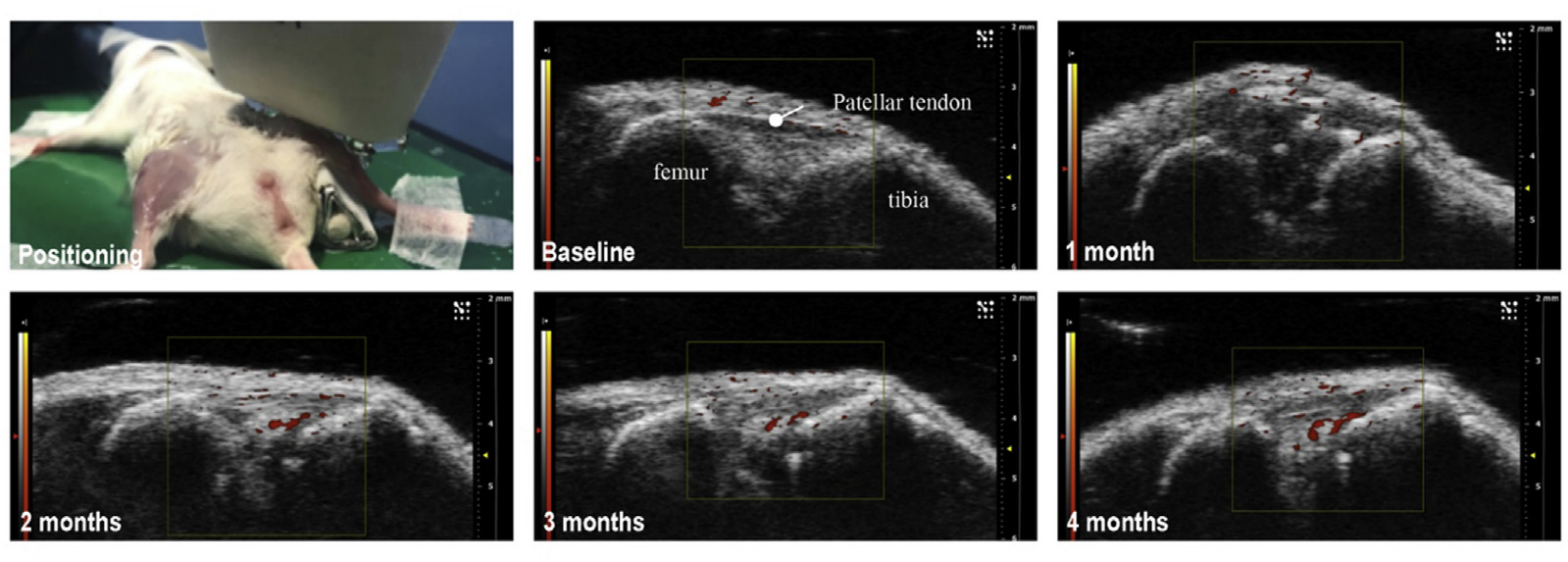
Ultrasound and Power Doppler imaging of intra-articular tissue damage and angiogenesis after destabilization of medial meniscus (DMM). Changes in intra-articular Doppler signals became evident by 2 months post DMM surgery (Wen, unpublished data). Similar findings are presented in^39^.
